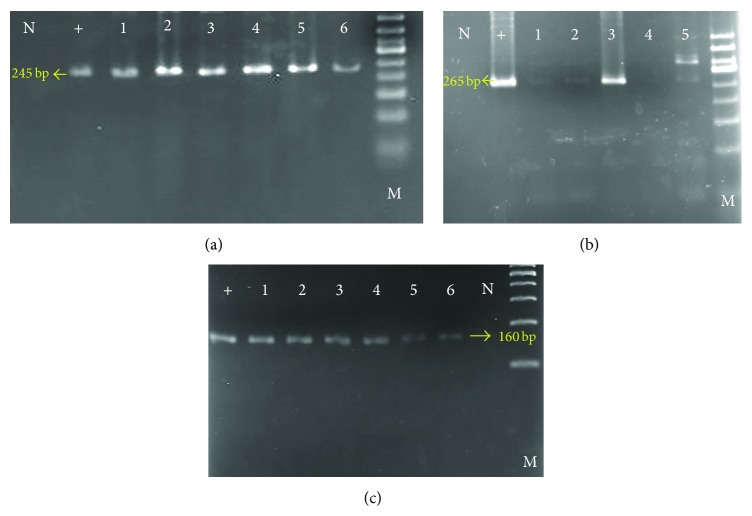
The figures show the result of RT-PCR analysis of positive mRNA expression of CTA genes in some patients. Each band represents a positive mRNA expression of CTA genes: (a), (b), and (c):* PAGE4*,* SCP-1*, and* SPANXA/D*, respectively. M: molecular marker; N: negative control; +: positive control.
